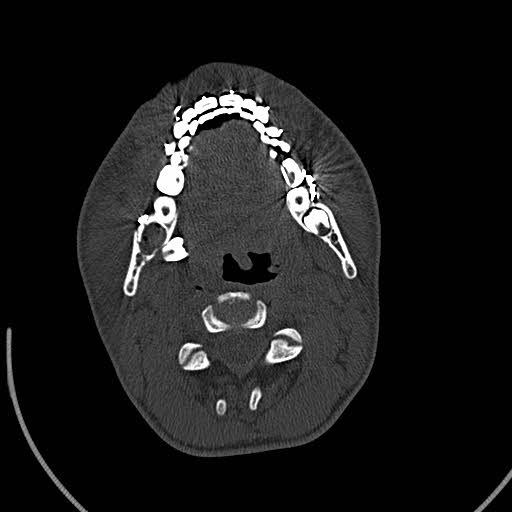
Computed tomography (CT) scan axial view. The third molar bud was displaced to the sublingual space
